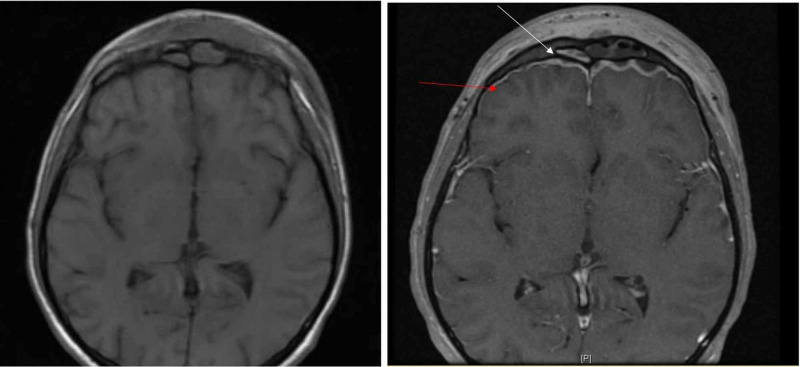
MRI T1-weighted pre- and post-contrast axials. Abscess is seen within the left frontal sinus (white arrow). Abscesses are seen underlying the bilateral frontal bones. Again seen is enhancement of the edematous frontal scalp compatible with Pott's puffy tumor. There is bilateral frontal pachymeningeal and leptomeningeal enhancement compatible with meningitis (red arrow).
